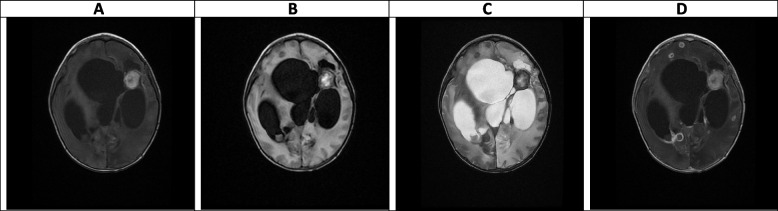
(the Brain MRI Without and with contrast, day 93). A-D Most of the mentioned findings in the previous study are seen without significant changes including abscess formation, hemorrhage, sign of ventriculitis, and associated hydrocephalus with midline shift
